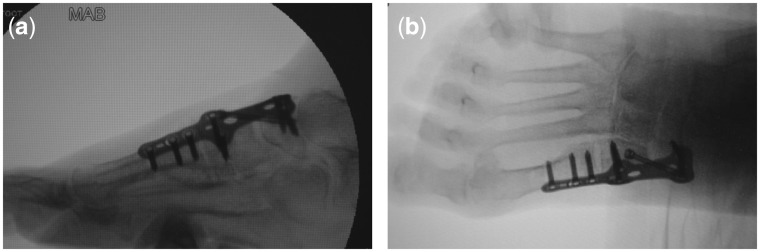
Transverse and sagittal section X-ray examination after surgical operation. Metatarsal-cuneiform joint and navicular-cuneiform joint of right lower extremity was perfectly stabilized with an internal fixation plate and screws shortly after surgical operation
